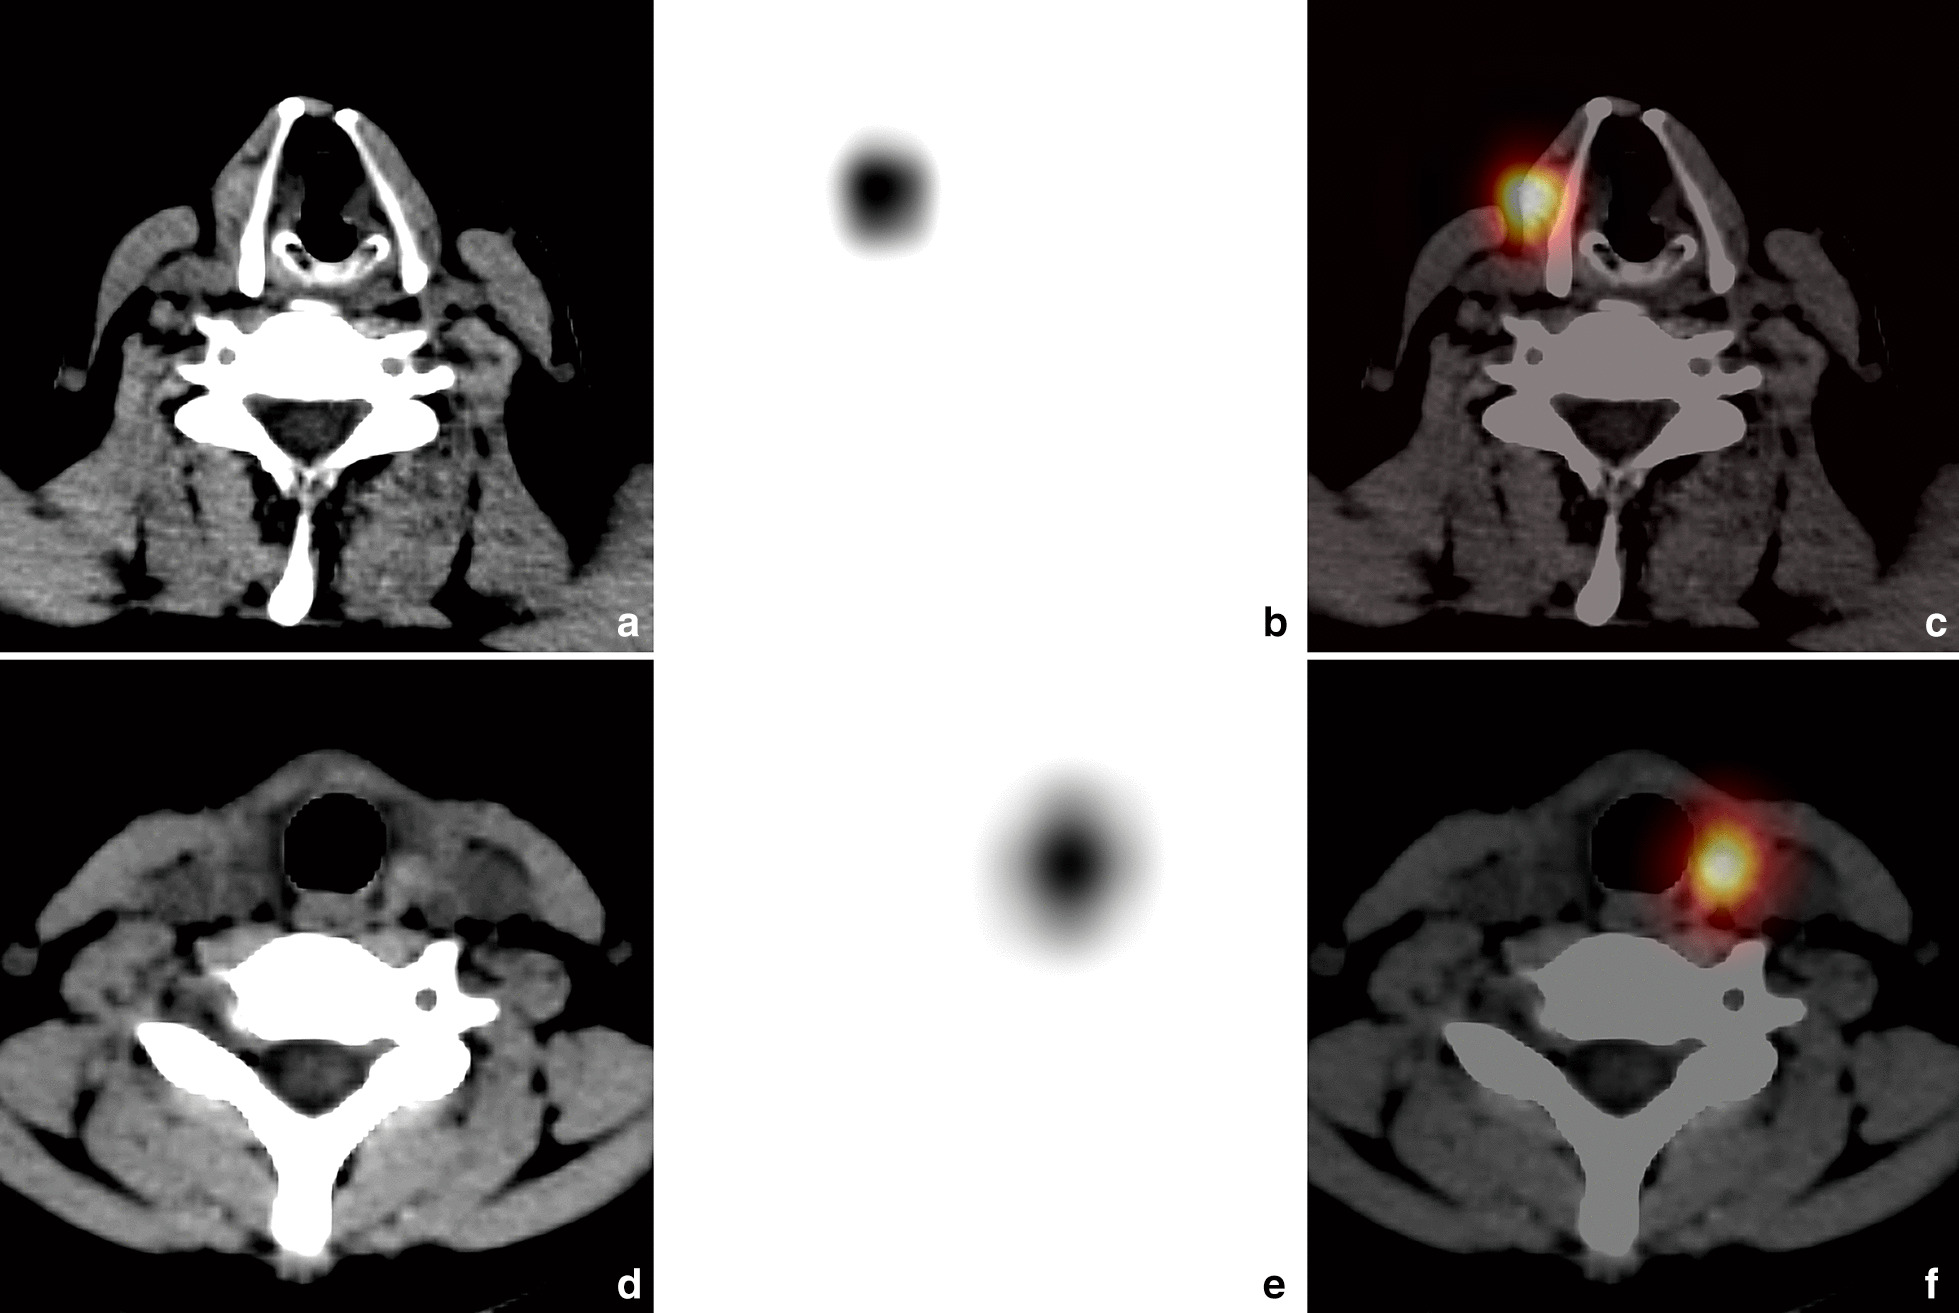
RTT lesions of type I (definite positive CT findings). The images (a CT; b SPECT; c fusion) were obtained from a 42-year-old man with PTC, who underwent total thyroidectomy 2 months ago. SPECT/CT demonstrated a RTT lesion with high-uptake and soft tissue nodule, adjacent to the thyroid right lamina (region IV). The images (d CT; e SPECT; f fusion) were obtained from a 15-year-old woman with FTC, who underwent total thyroidectomy 2 months ago. SPECT/CT revealed a RTT lesion high-uptake and soft tissue nodule, adjacent to the left side of tracheal cartilage (region I)
